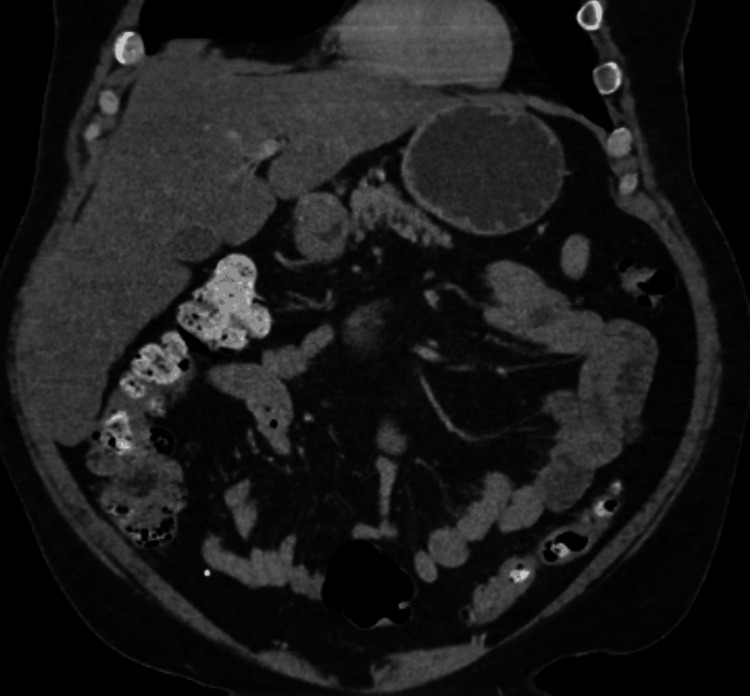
CT abdomen pelvis at admission (coronal view) with no acute abnormality
